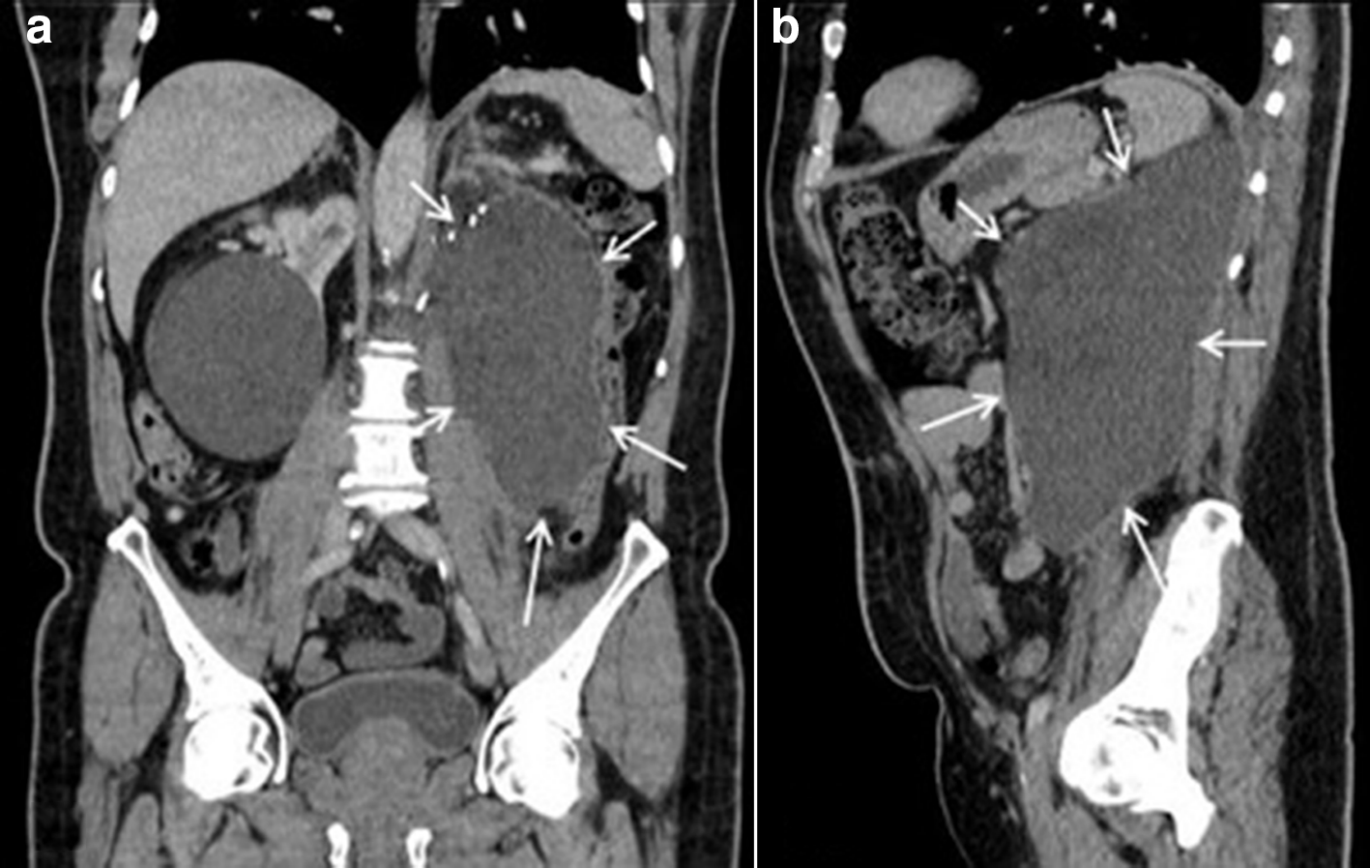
Coronal (2a) and sagittal (2b) slices of the CT abdomen and pelvis showing
